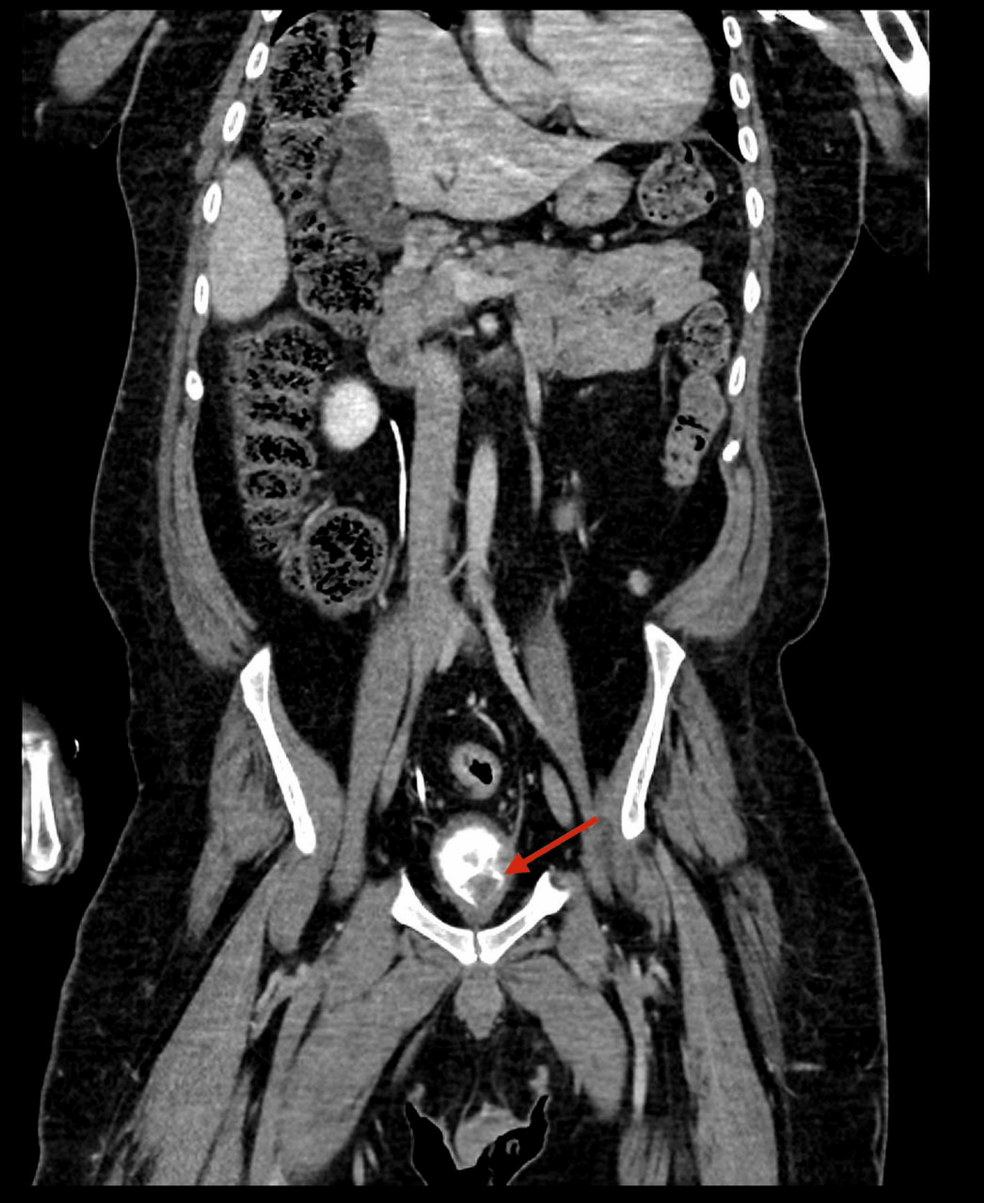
CT urography (coronal view) showing a bladder tumor (arrow) on the left lateral wall. The mass enhances irregularly and protrudes into the bladder cavity, creating a filling defect and distorting the bladder outline. Abnormal finding: irregular intravesical mass. Normal findings: no hydronephrosis, no upper tract involvement, and no spread outside the bladder.
